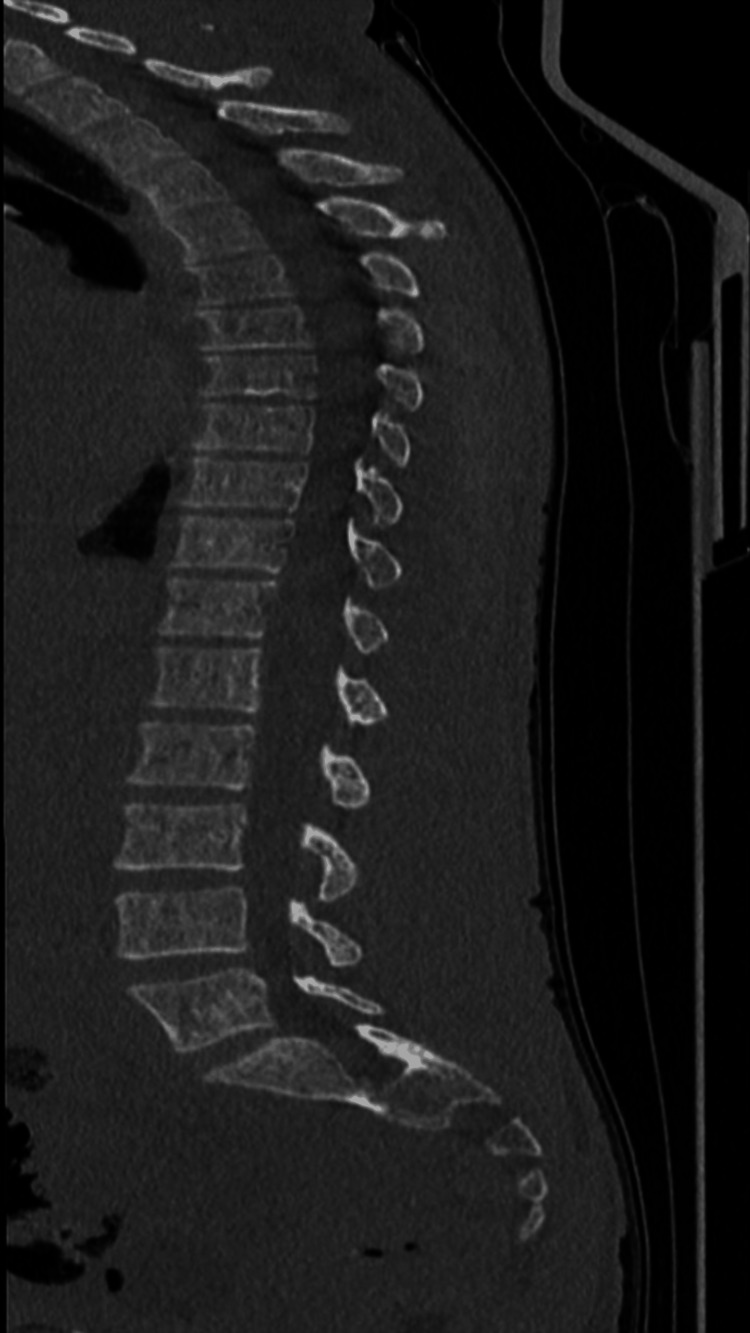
Spine CT scan of the first patient (FR) This sagittal CT image of the spine demonstrates a normal osseous anatomy from the thoracic spine through the sacrum. The vertebral bodies are well-aligned, with preserved vertebral height and intact cortical margins, showing no signs of compression fractures or lytic lesions. The intervertebral disc spaces are maintained throughout, suggesting the absence of significant degenerative disc disease. CT: computed tomography
